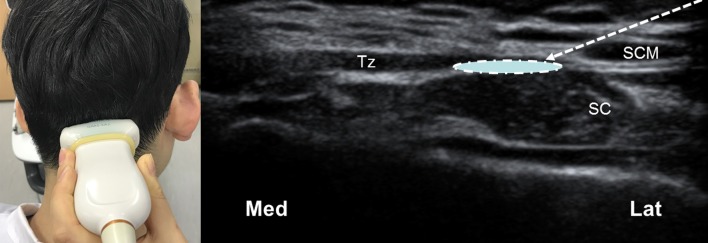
Another suggested injection point for GON blockade with ultrasound guidance. The linear transducer (L12-5 MHz, HDI 5000, Philips, USA) is positioned to allow a short-axis view. The arrow and circle indicate the needle trajectory and the target point for spreading local anasthetics, respectively. Med, medial side; Lat, lateral side; SC, semispinalis capitis muscle.
